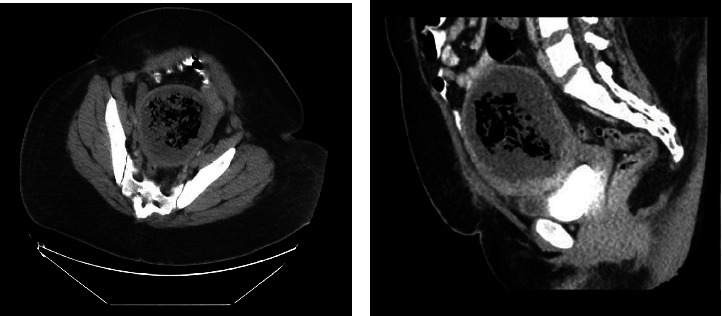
CT scan of the pelvis showing (a) transverse and (b) sagittal views of the uterus with internal foci of gas representing areas of necrosis.
